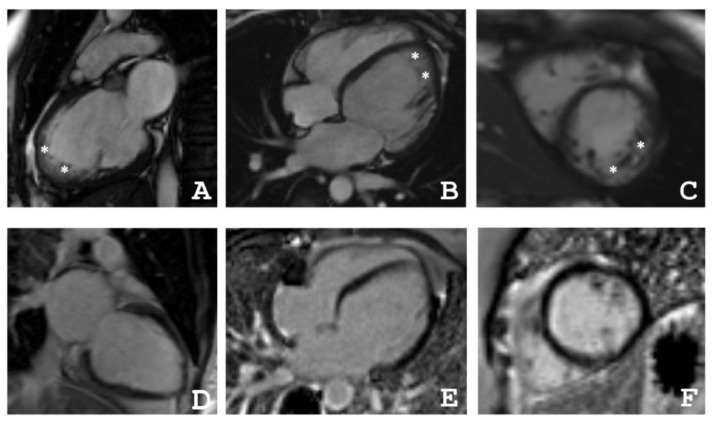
(A–C) Proband’s (III-4) cardiac magnetic resonance imaging in movie mode, steady-state free-precession (SSFP) sequence: (A) long axis 2–chamber projection, (B) long axis 4–chamber projection, (C) short axis at the level of the apical segments, * indicates a layer of non-compacted myocardium, (D–F) delayed contrast. Inversion recovery sequence with suppression of the signal from the myocardium. No areas of contrast, which indicates the absence of areas of intramyocardial fibrosis, scarring, and inflammatory myocardial damage.
